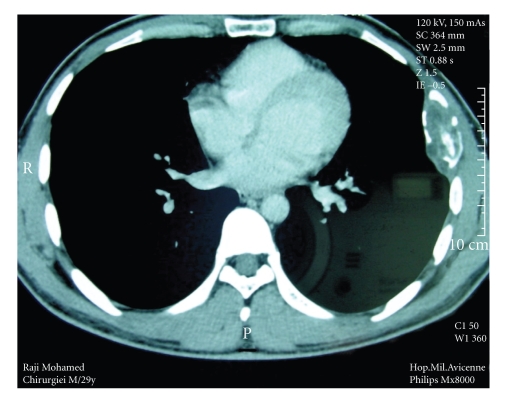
Chest CT scan: tumor of the 6th left rib without invasion of adjacent tissues.
